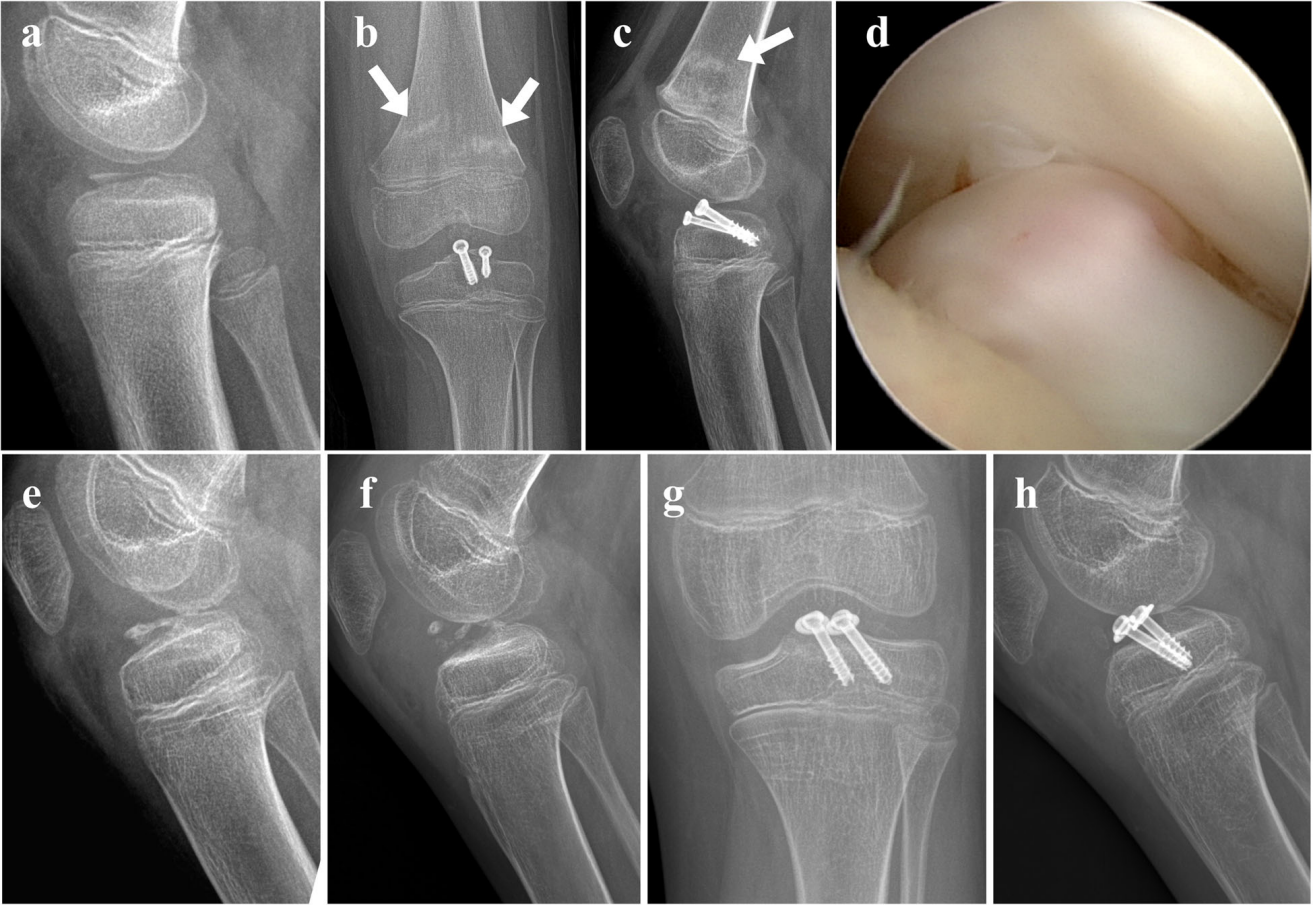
a A 8–9 year-old boy visited the clinic at 32 days post trauma with type III tibial eminence fracture. b, c Radiographs taken at postoperative 2.5 months. Incomplete distal femoral metaphyseal fracture (arrow) occurred during vigorous physical therapy. d While removing screws, it was found that the head of 5.0 mm screw impinged against the lateral portion of the medial femoral condyle and caused extension deficit of the knee. e Radiolucent gap between avulsed fragment and host bone was found on the day of screw removal. f A radiograph taken at postoperative 18 months. He was lost to follow-up for 1 year. He returned to the clinic with flexion contracture of 25° and re-displaced avulsed fragment. g, h He underwent open reduction and internal fixation with two 5.0 mm screws in conjunction with two washers for re-displaced fragments
